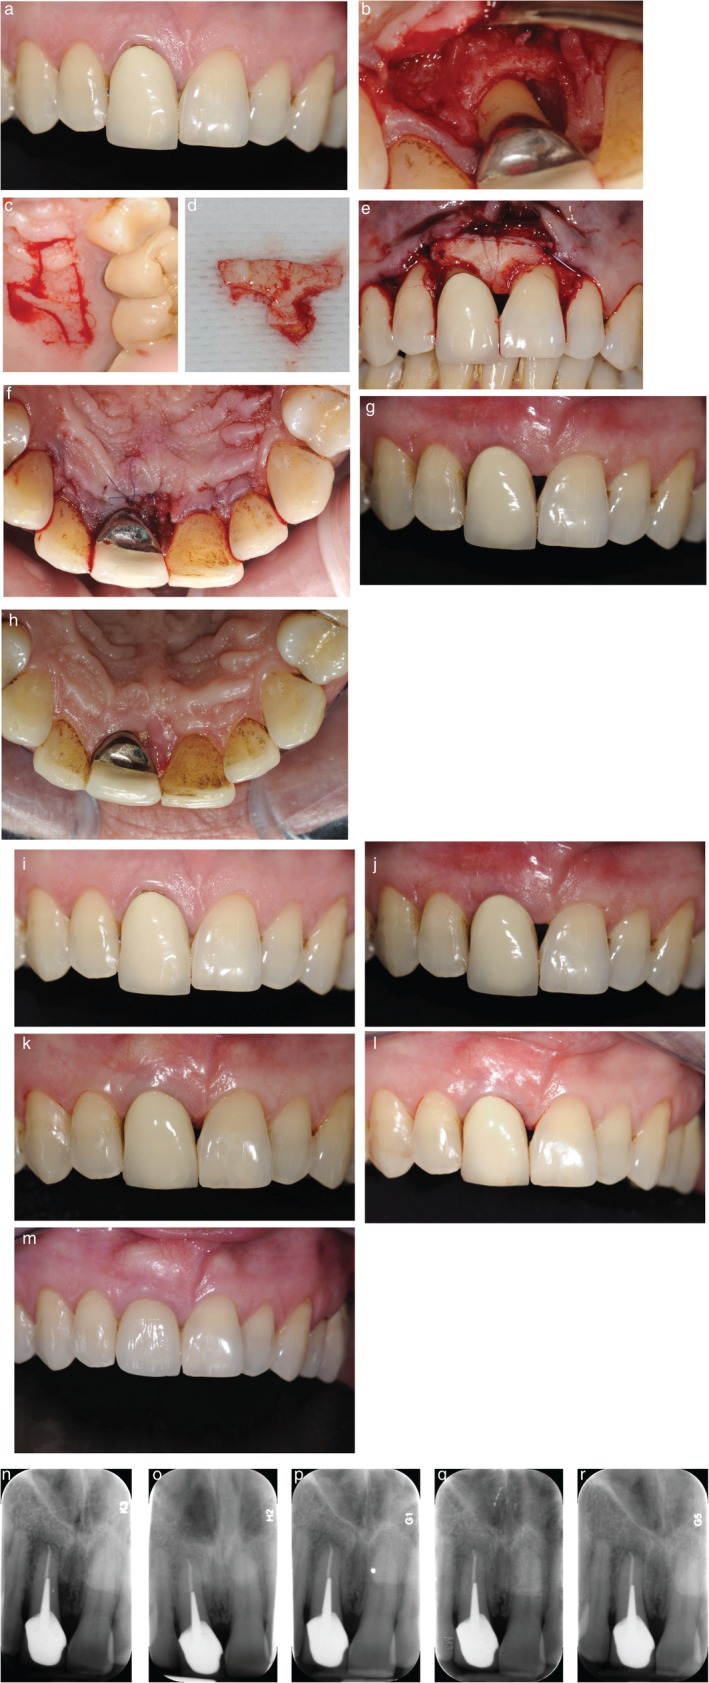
Case 2 (a) Preoperative view showing prosthetic crown malposition and minor papillary deficiency. Infrabony defect after degranulation (b); harvesting of a T‐shaped palatal graft (c, d); CTG placement with additional compressive suture to enhance graft stability (e). (f) Immediate postoperative view showing primary closure. (g, h) Postoperative views at 15 days on the buccal and palatal aspects, showing maintained closure and residual black triangle. (i–m) Soft tissue maturation from baseline to 6, 12, and 55 months; the prosthetic crown was replaced at 24 months. (n–r) Radiographic follow‐up from baseline to 6, 12, 24, and 55 months showing bone fill and long‐term stability.
